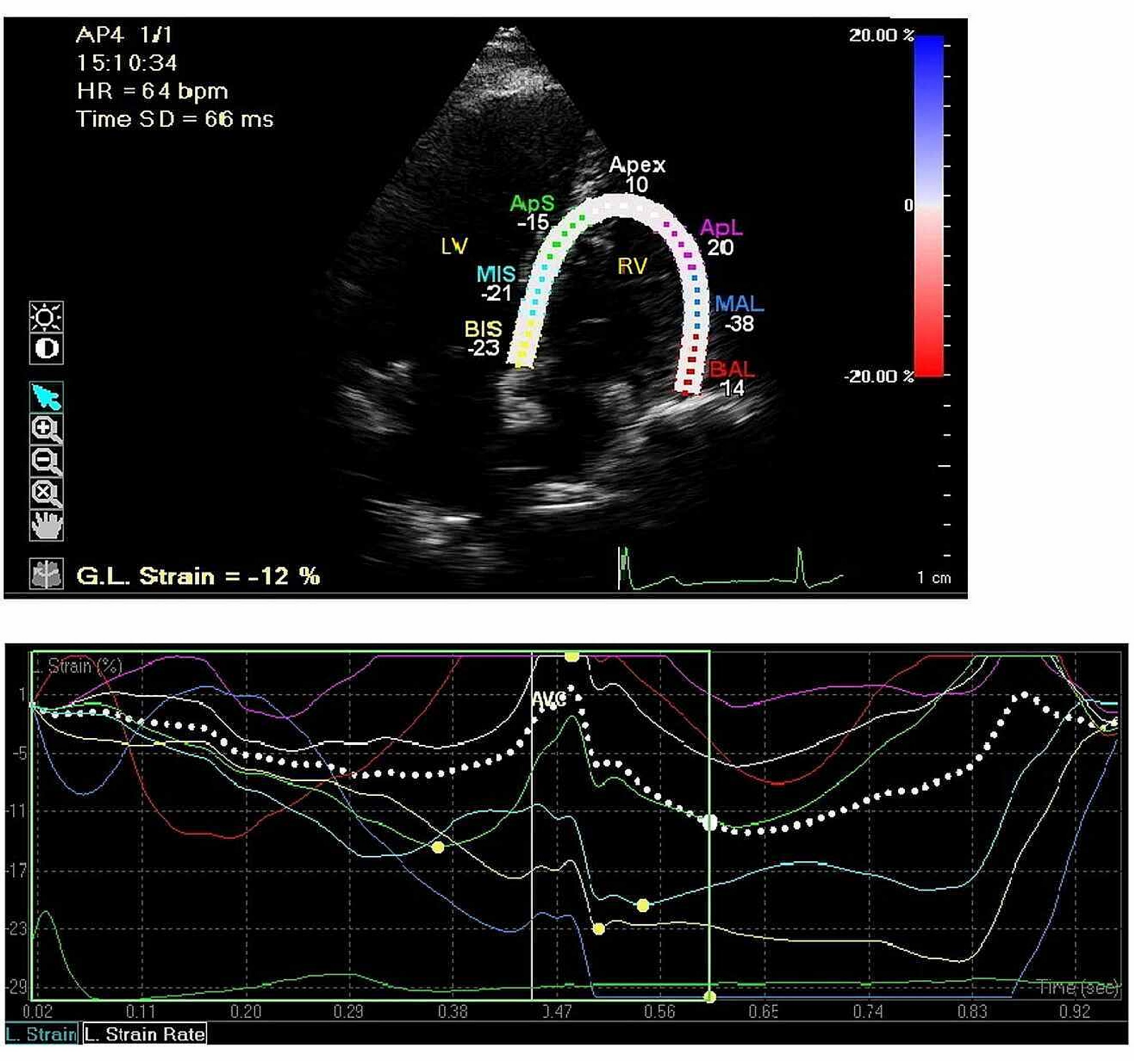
Longitudinal strain (LS) in an apical four-chamber view. Right side average segmental strain displayed in graphs.
